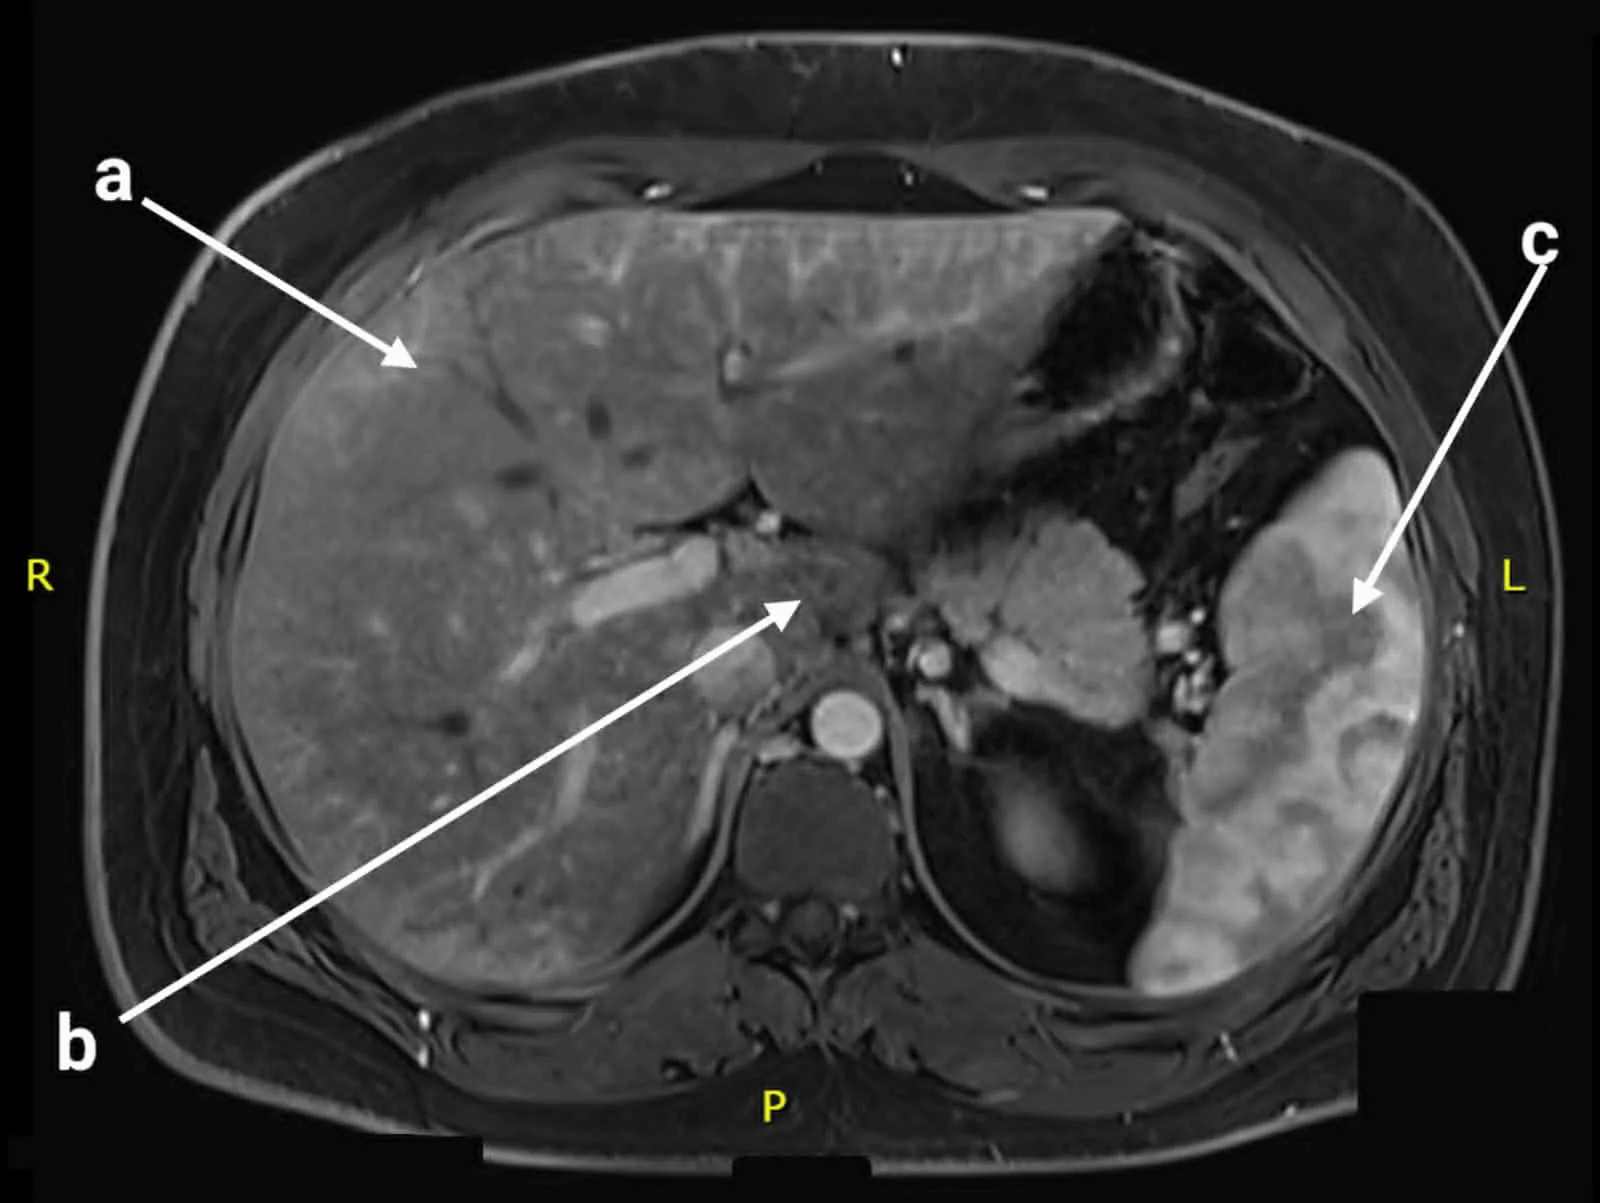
Contrast-enhanced magnetic resonance imaging of the upper abdomen Axial contrast-enhanced magnetic resonance imaging demonstrates (a) an enlarged, edematous liver, (b) enlarged porta hepatis lymph nodes, and (c) splenomegaly. Magnetic resonance cholangiopancreatography showed no biliary duct dilation or choledocholithiasis.
